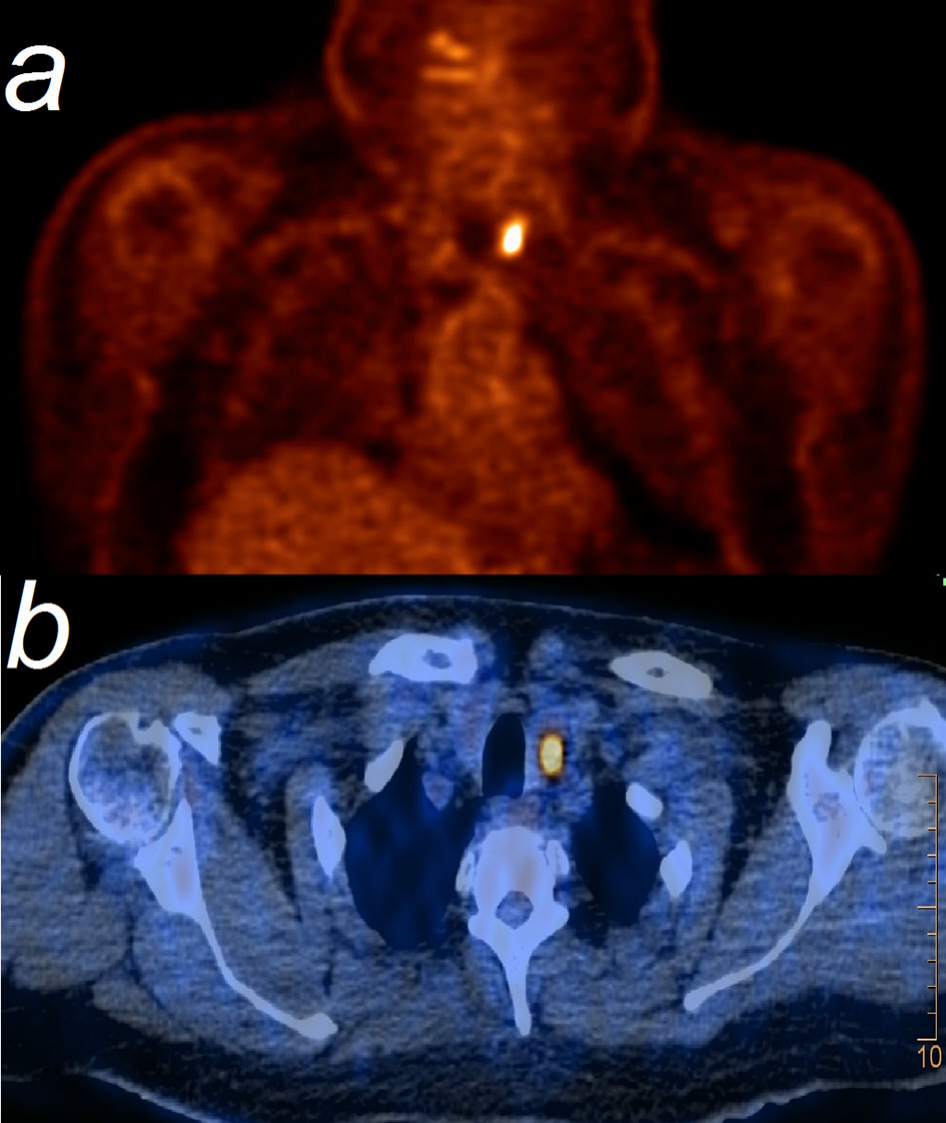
FDG-PET (A) shows a marked focal uptake with an SUV=14, corresponding to left thyroid lobe at PET/CT (B).
